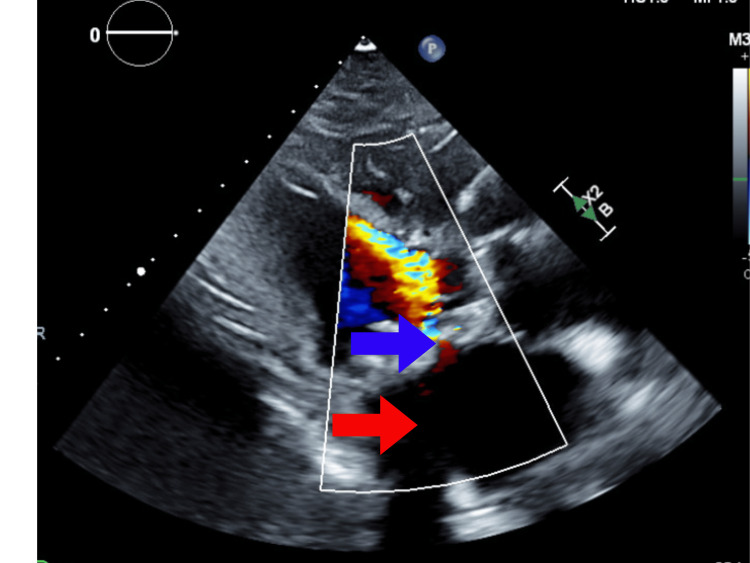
Preoperative echocardiogram showing mitral valve stenosis (blue arrow) and dilated left atrium (red arrow).
